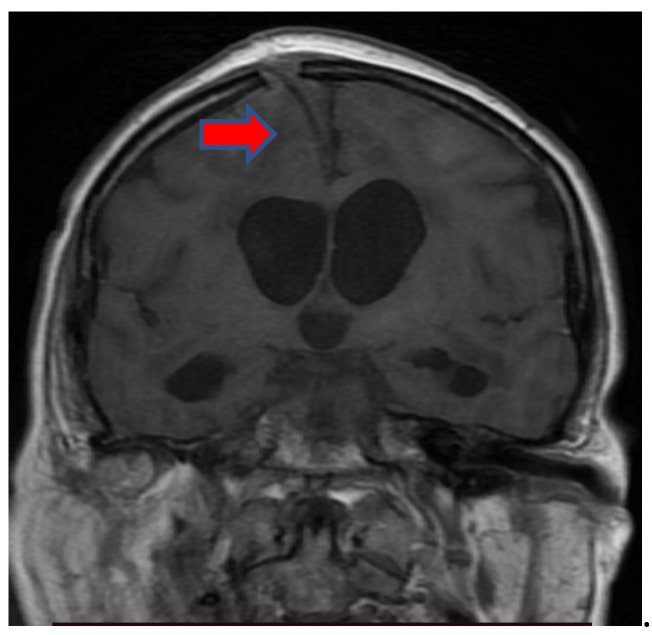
MRI coronal T1-weighted sequence noting secondary hydrocephalus that appeared as a complication of encephalitis, for which external drainage was installed (red arrow).
